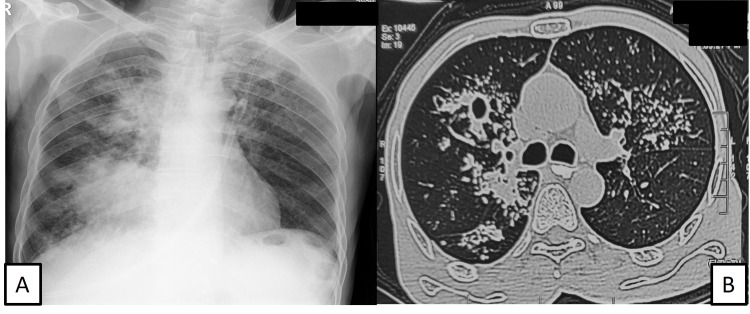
(A) Chest radiograph showing right upper zone and middle zone consolidation. (B) Chest CT showing bilateral upper lobe centrilobular nodules and right upper lobe cavitation with consolidation.
